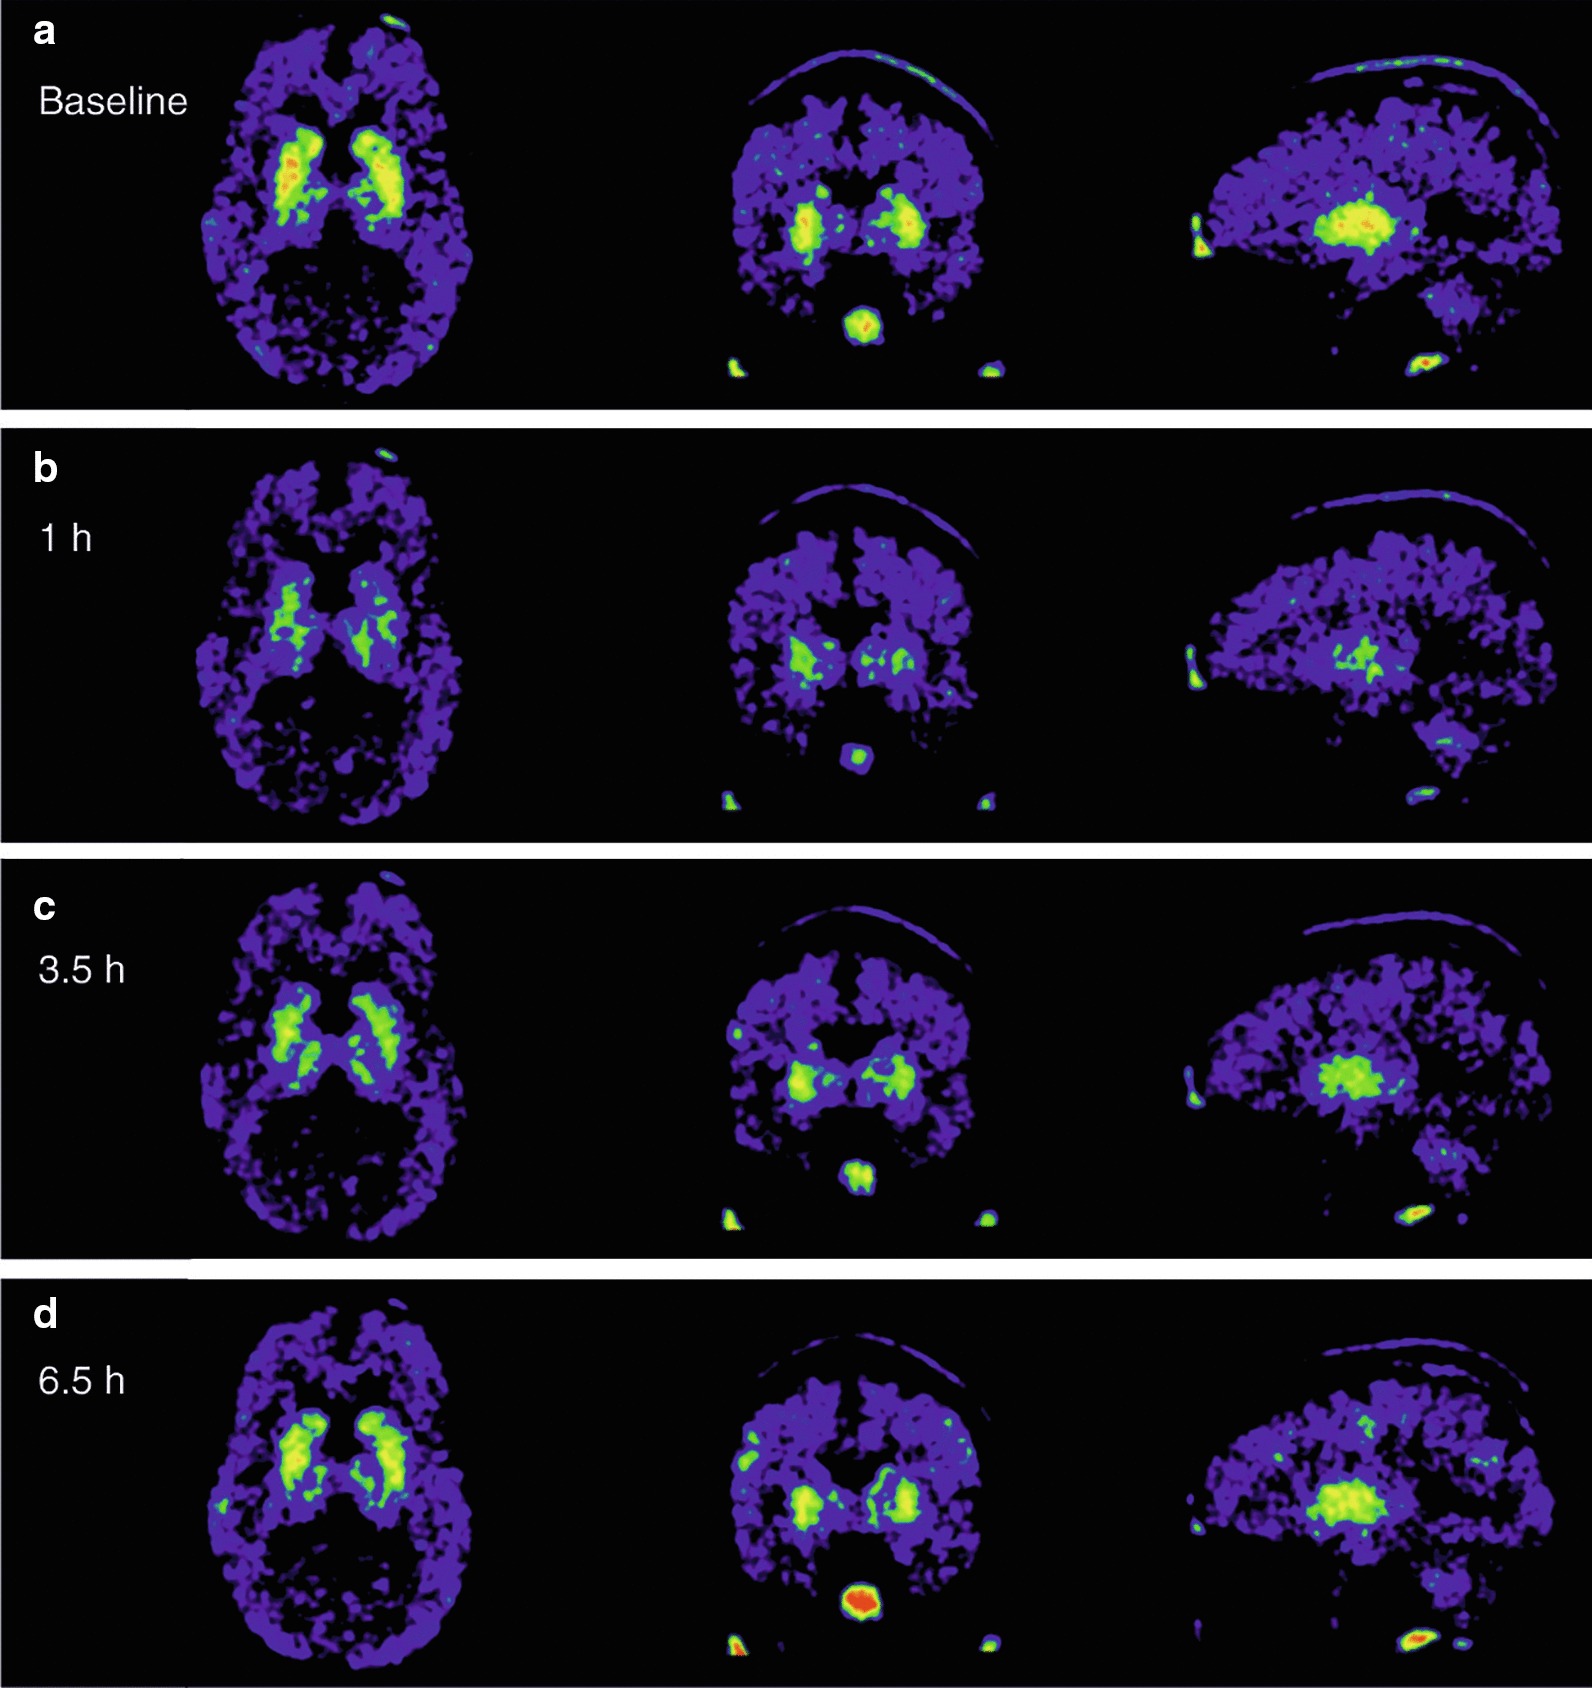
A representative set of human brain PET SUV images from one healthy male volunteer at baseline and 1 h, 3.5 h and 6.5 h after 60 mg of ORM-12741
